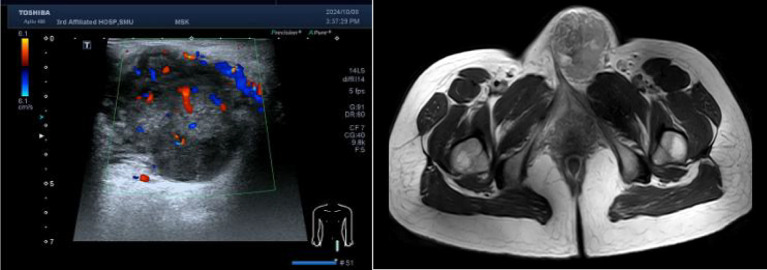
Left: Real-time ultrasonogram of the lesion. Right: Magnetic resonance imaging of the pelvic.
